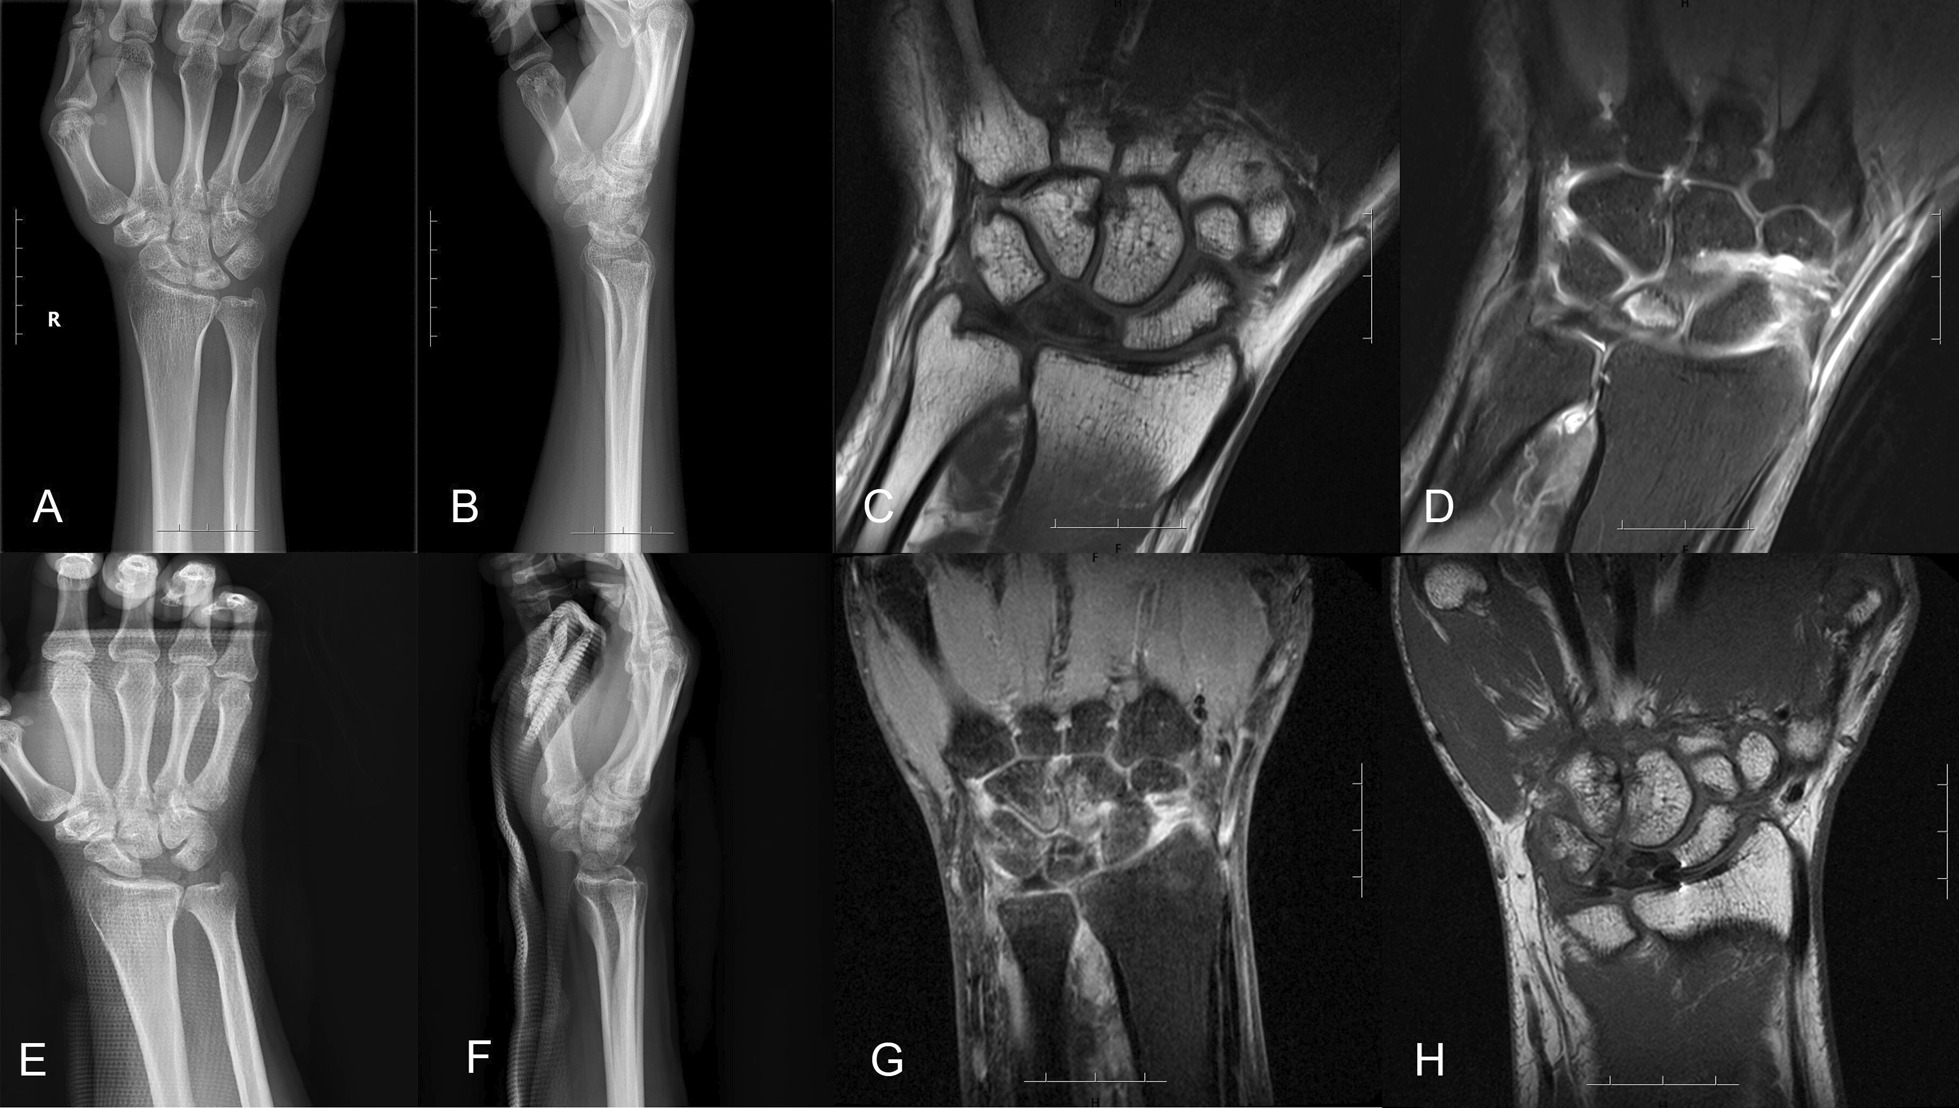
A typical case of stage IIIb of KD in a 40-year-old man in his right wrist, who had a disease course of 6 months, underwent the removal of necrotic lunate and palmaris longus tendon ball arthroplasty. The preoperative radiographs (A and B) and MRI findings (C and D) showed the KD in stage IIIb, and postoperative radiographs (E and F) and MRI findings (G and H) showed palmaris longus tendon ball interposition in place of removed necrotic lunate
